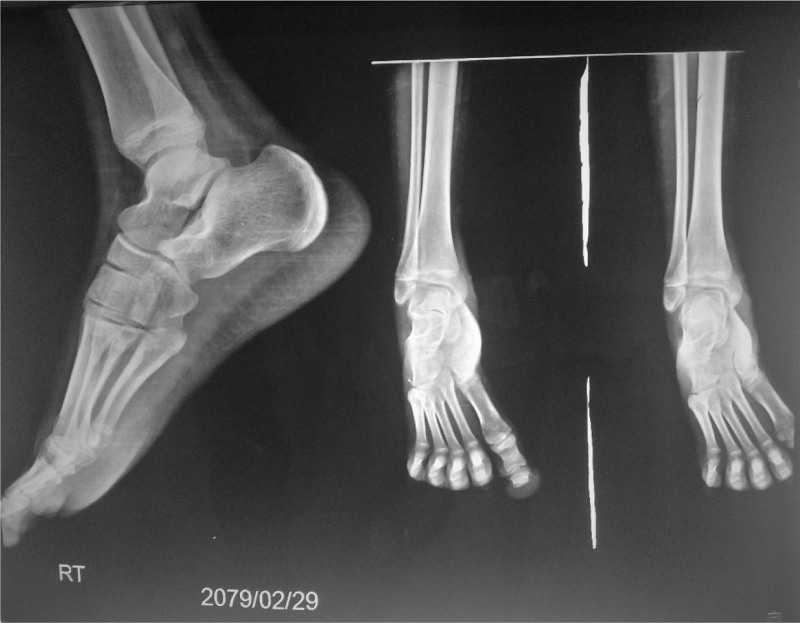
Normal lower foot X-ray.
